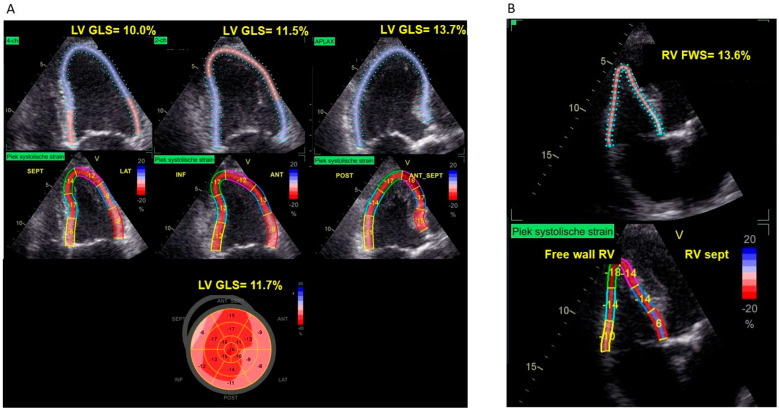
Example of LV GLS and RV FWS measurement in a patient with severe AS who died during follow up. (A) Echocardiographic images of LV GLS from four-, two-, and three-chamber views with bull’s eye plot. The bull’s eye plot demonstrates an impairment of LV GLS (11.7%), particularly of the basal LV segments. (B) Echocardiographic images of RV FWS from the RV-focused apical four-chamber view. The absolute value of 13.6% of RV FWS demonstrates an impaired RV FWS. LV: left ventricle; LV GLS: LV global longitudinal strain; RV: right ventricle; RV FWS: RV free wall strain; AS: aortic stenosis. Strain values are expressed as absolute values. 4-ch: 4-chamber view; 2-ch: 2-chamber view; APLAX: apical long axis view. TAPSE: tricuspid annular plane systolic excursion; ANT: anterior; ANT_SEPT: antero-septal; INF: inferior; LAT: lateral; POST: posterior; SEPT: septal.
